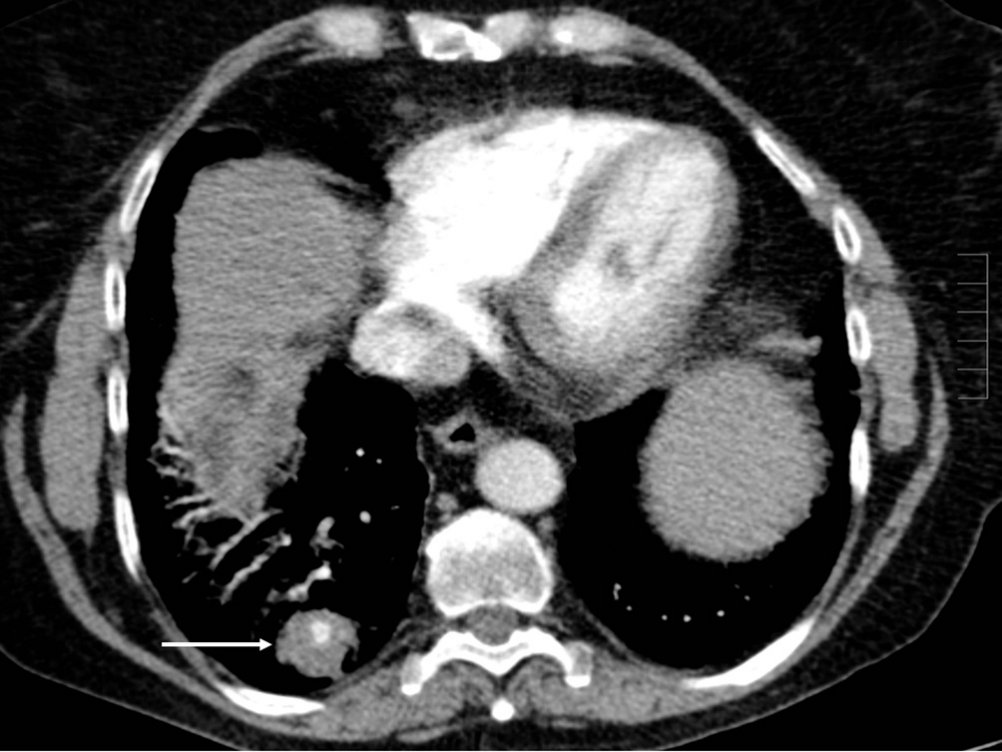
Axial contrast-enhanced CT scan of the chest in soft tissue window demonstrating a right lower lobe solid nodule (arrow) with central calcified focus.
